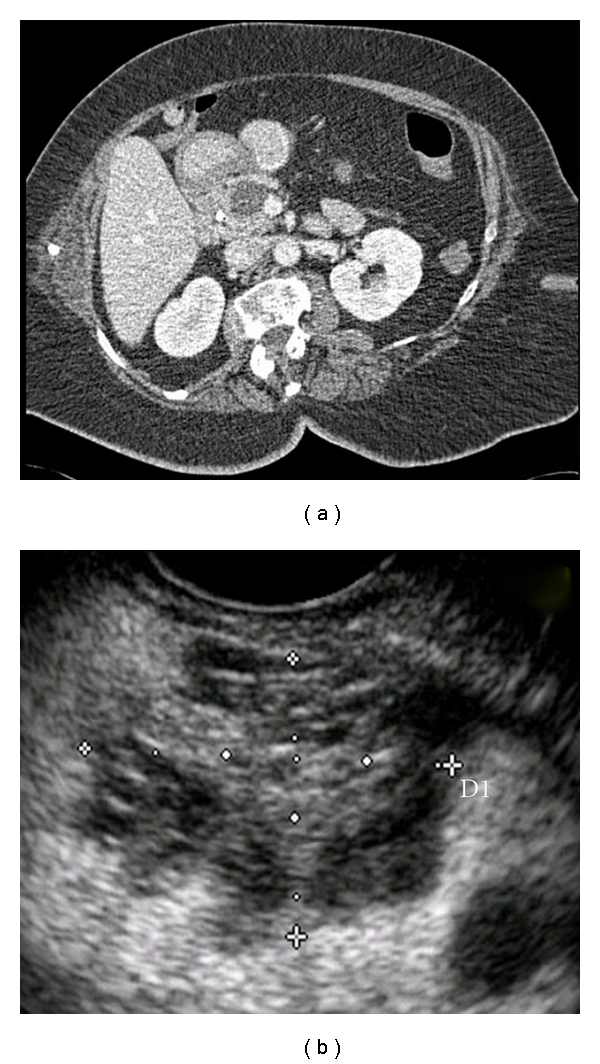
Serous cystic neoplasm: (a) CT and (b) EUS images both showing the central starburst calcification pattern characteristic of serous cystic neoplasms.
